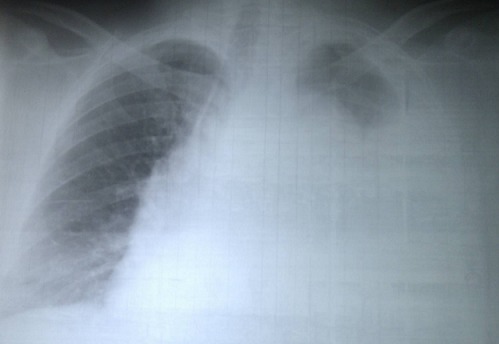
Radiographie du thorax à l'admission en cardiologie
